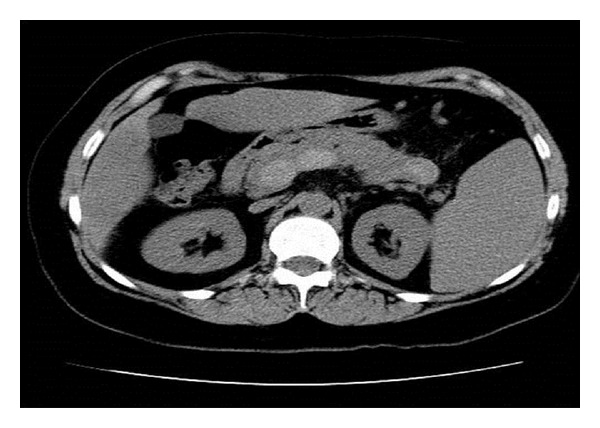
CT demonstrating splenic thrombosis.
